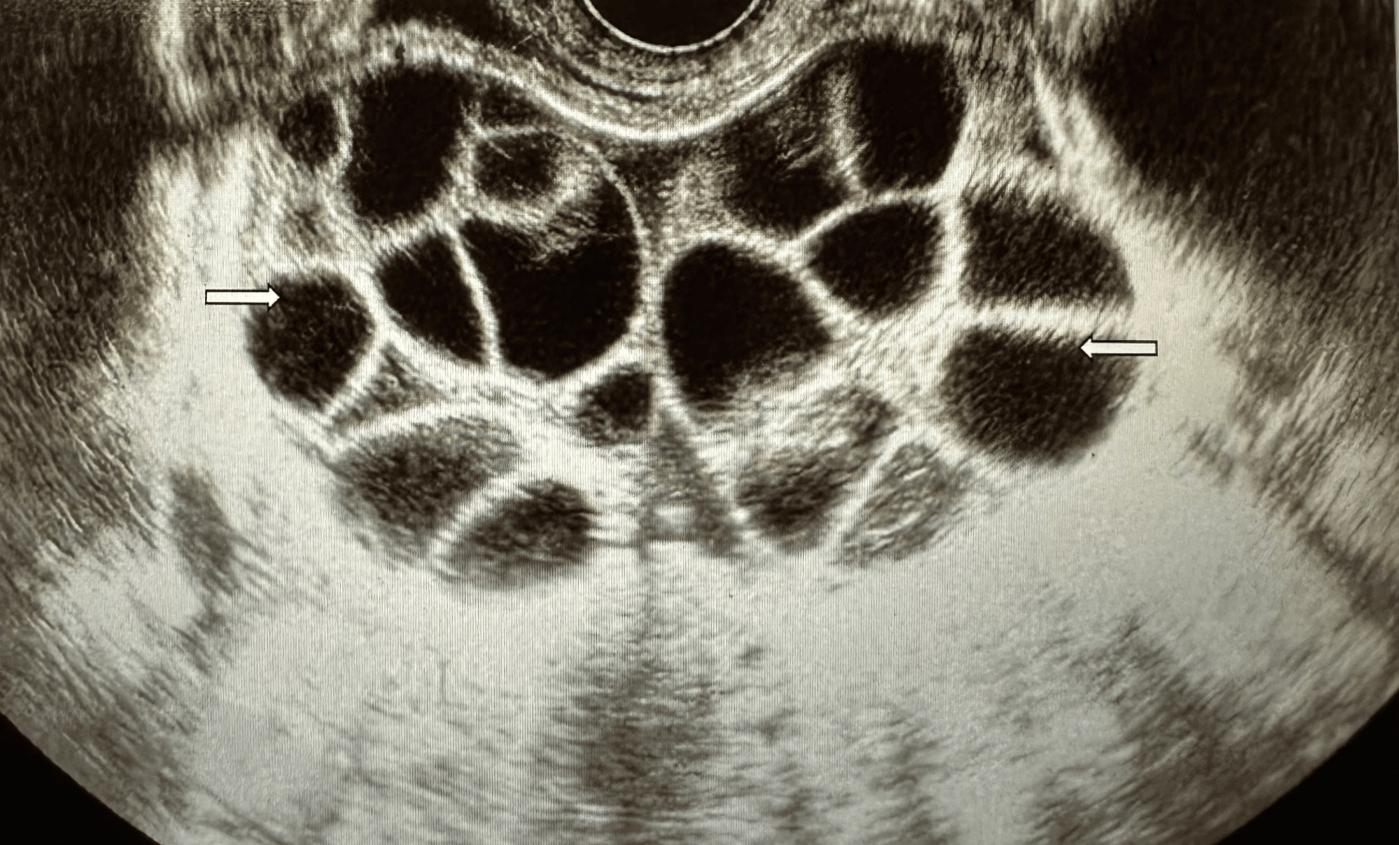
Ovarian Cysts Associated With Theca Lutein Hyper-Stimulation Ultrasound image showing bilateral ovarian enlargement with multiple cysts (white arrows), consistent with theca lutein cysts secondary to elevated β-hCG in molar pregnancy. The right ovary measured 108 × 73 mm, and the left ovary measured 84 × 75 mm. This finding reflects ovarian hyperstimulation associated with high β-hCG levels and is a recognized complication of complete hydatidiform mole.
